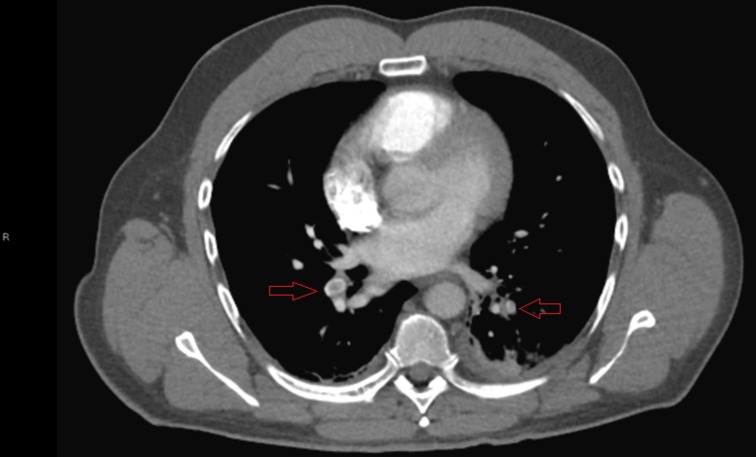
Computed tomography angiography demonstrating thrombi in lower lobe segmental arteries bilaterally
